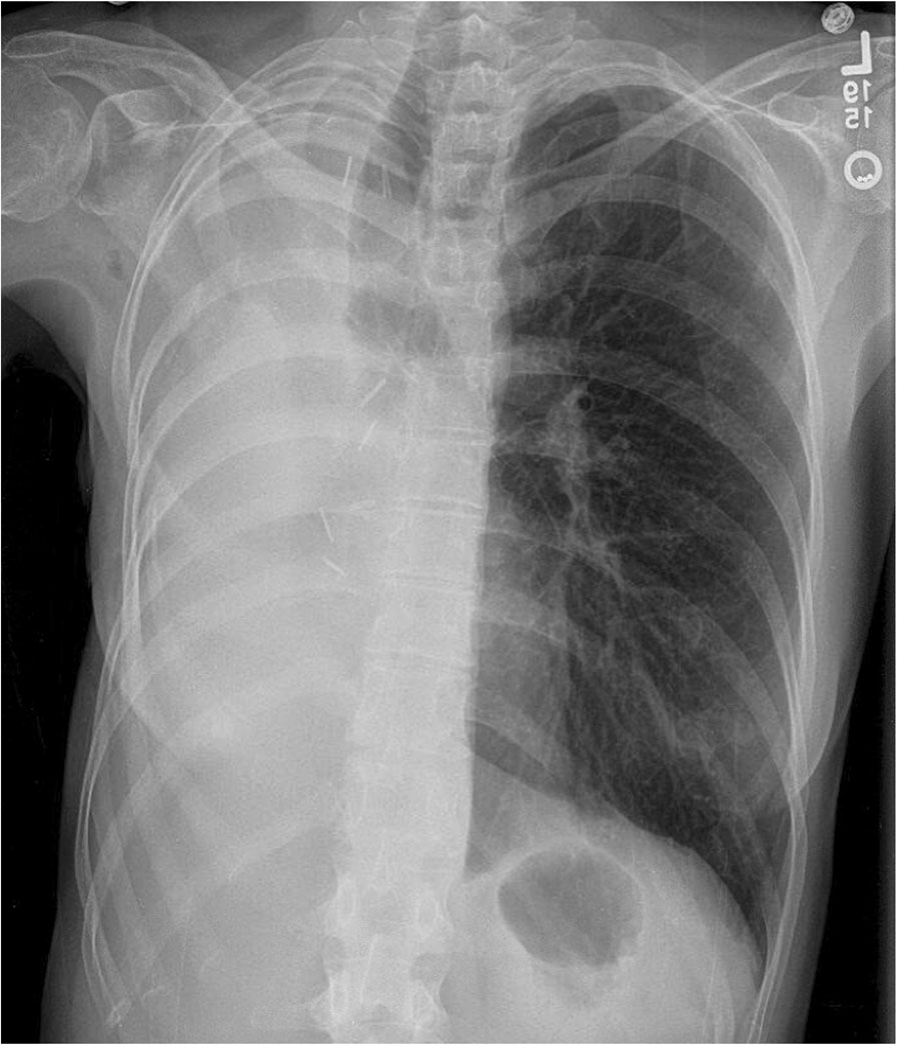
Chest radiograph four weeks after placement of saline implants shows medialization of the inferior mediastinum and residual rightward tracheal deviation
